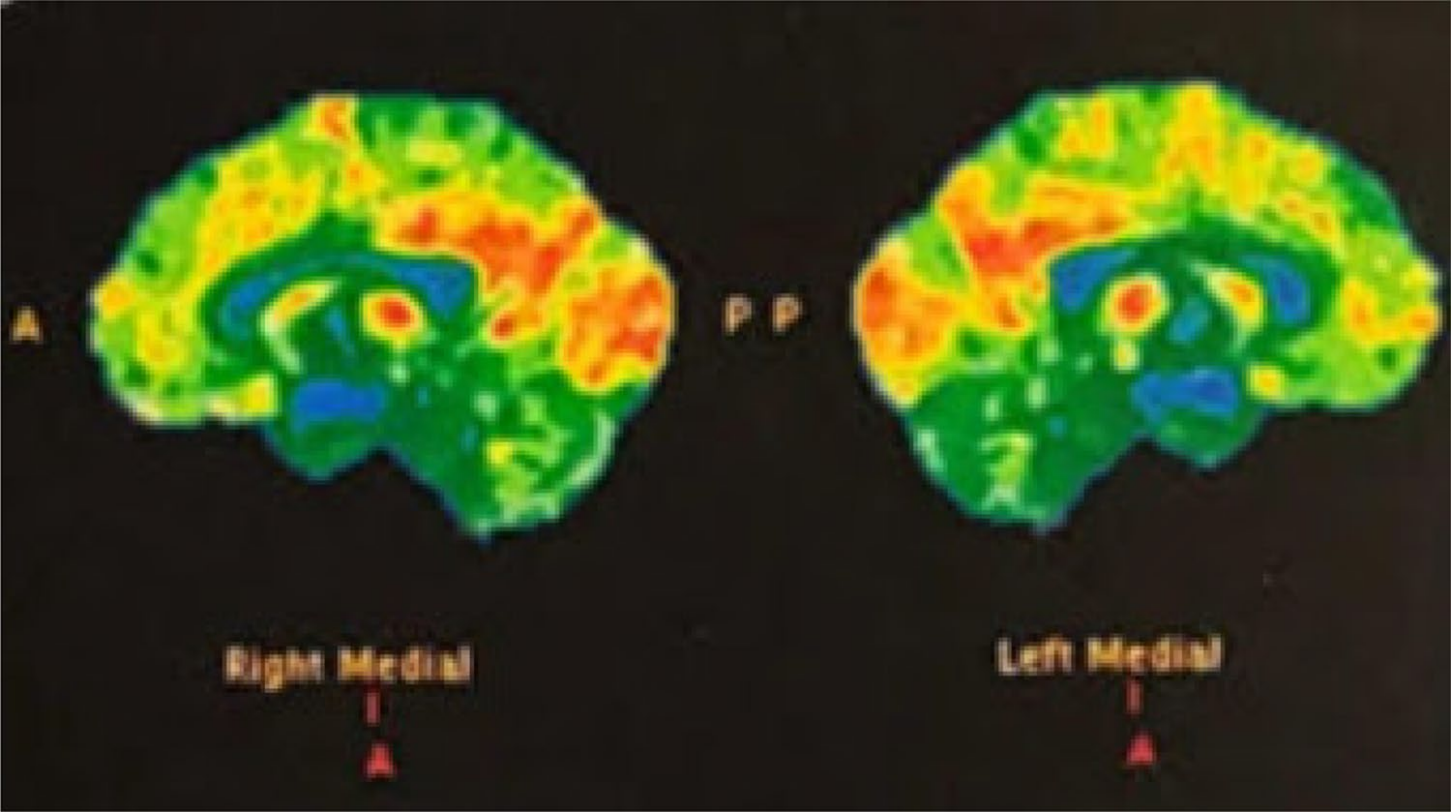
Brain ^18^F-FDG PET findings: hypometabolism in the medial frontal cortex
